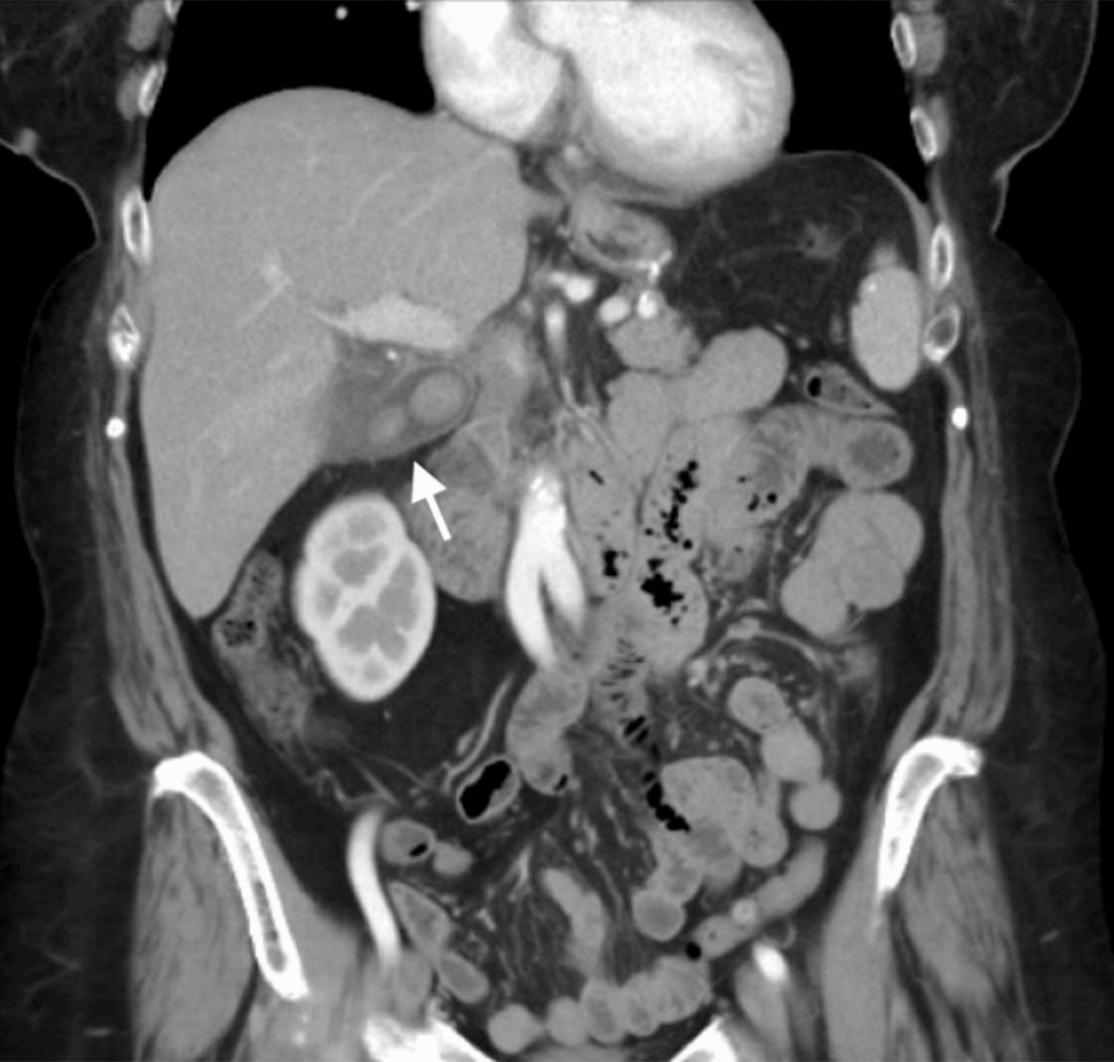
Coronal CT image showing recurrent acute calculous cholecystitis The white arrow indicates a gallbladder remnant that is distended with cholelithiasis, wall thickening, and pericholecystic fluid.
